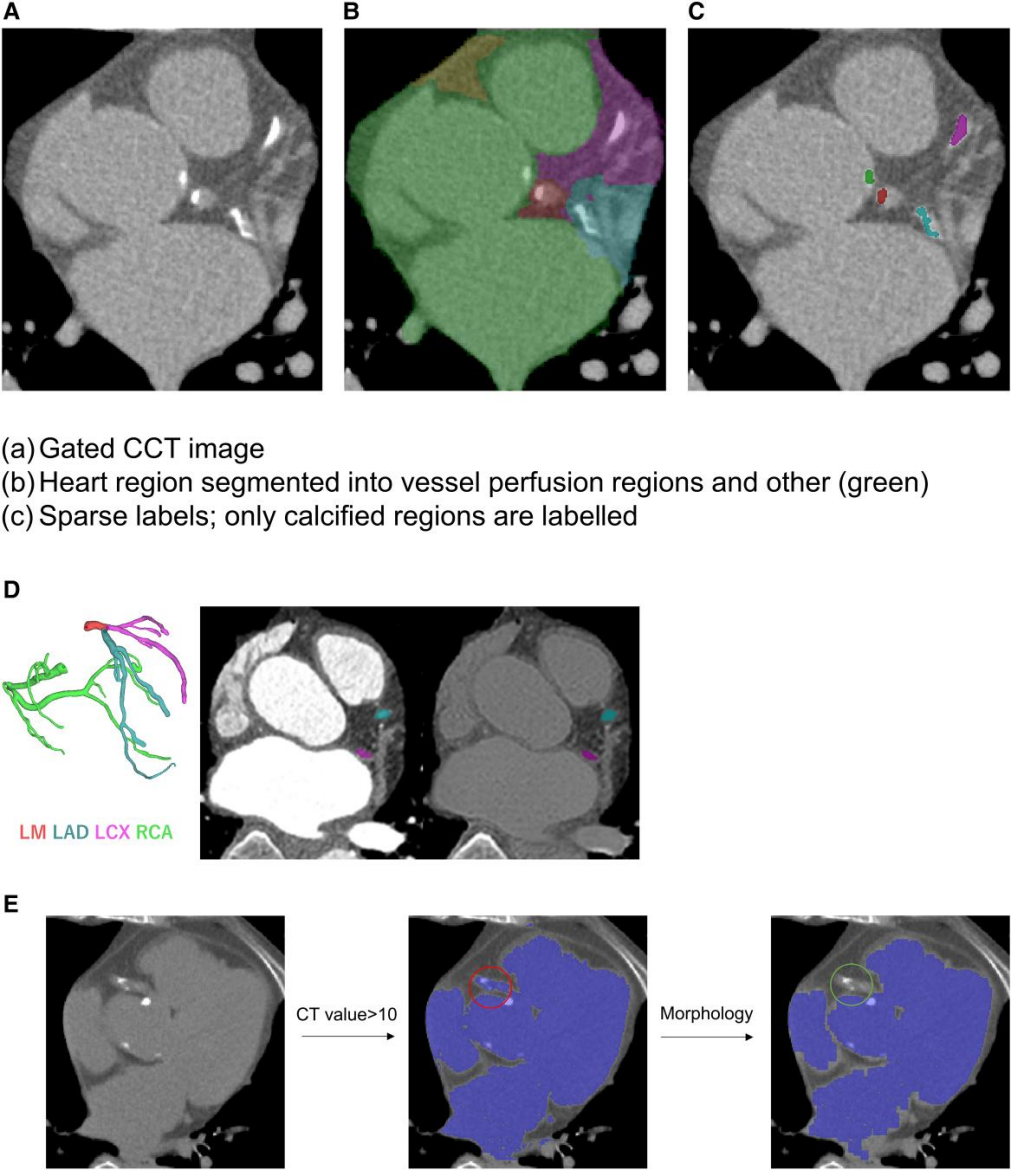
Heart-labelling method. (*A*) Gated CCT image. Calcifications are localized in LM, LAD, LCX and another (aorta) region. (*B*) Heart labelling into vessel perfusion regions and other region (green). (*C*) Sparse labels; only calcified regions are labelled. (*D*) Coronary labels. In 3D coronary CT angiography, whole coronary arteries are labelled (left). The synthetic image is generated by transforming contrast enhanced image (mid) into non-contrast image (right). (*E*) Non-coronary labels. Non-coronary label generation. Original CCT image (left), labelling by CT value threshold (middle), removal of the coronary artery region by applying multiple erosion operations followed by dilation which removes any thin tube-like structures (right).
